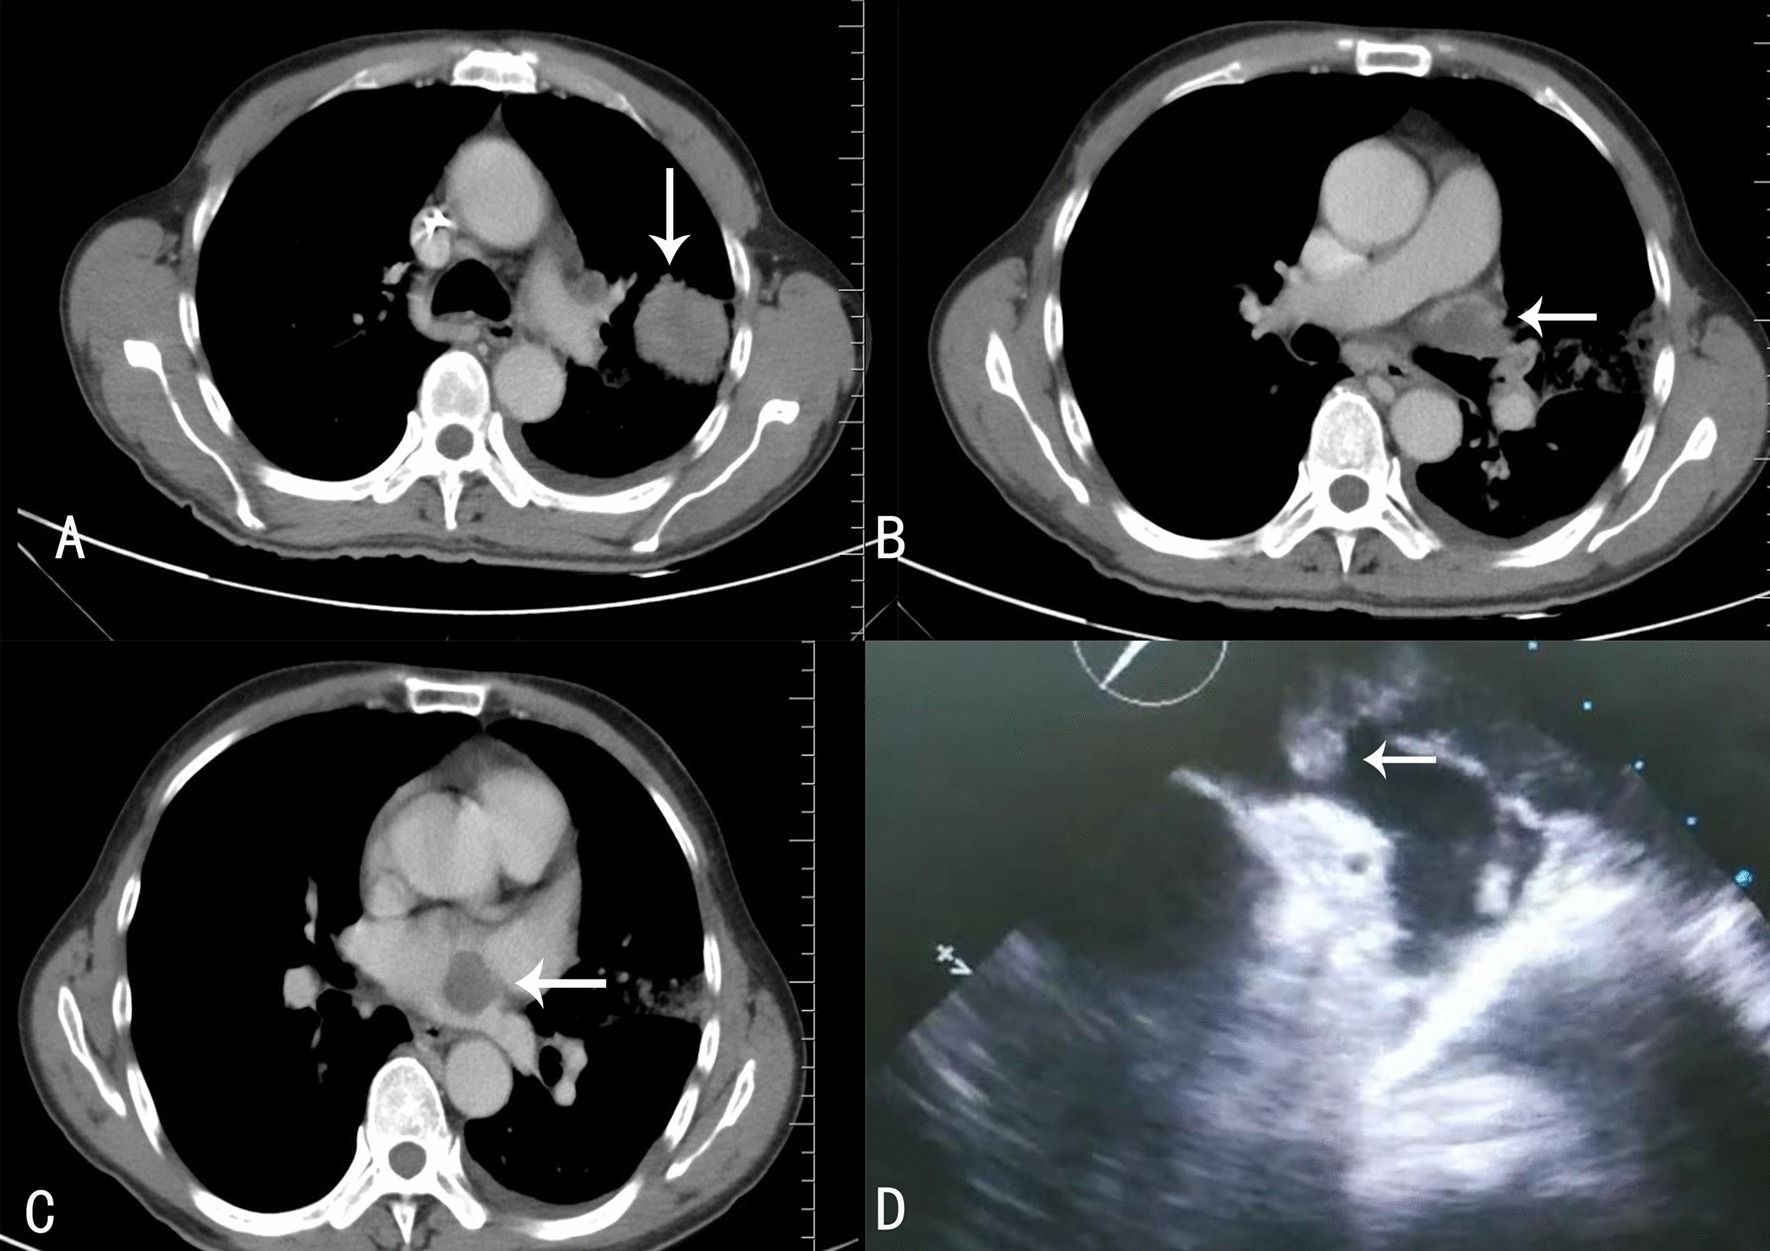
Chest computed tomography (CT) scan and transesophageal echocardiography. a Solid lesion with irregular borders in the upper left lobe of the lung (white arrow). b The upper left pulmonary vein was occluded by solid material(white arrow). c Solid mass in the left atrium(white arrow). d Transesophageal echocardiography revealed a solid mass in the left atrium growing from the entrance of the left superior pulmonary vein (white arrow)
